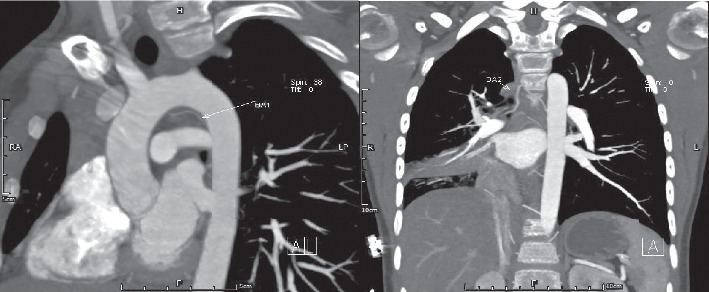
CTA demonstrated that BA1 originated from the thoracic aorta to the left at the level of the upper margin of T5 and entered the right hilum above the bifurcation of the trachea. BA2 arose from the right side of the thoracic aorta at T6 and passed up to the right and then down to the right hilum, with a widening of the diameter (2 mm) of the primary and middle tubes.
